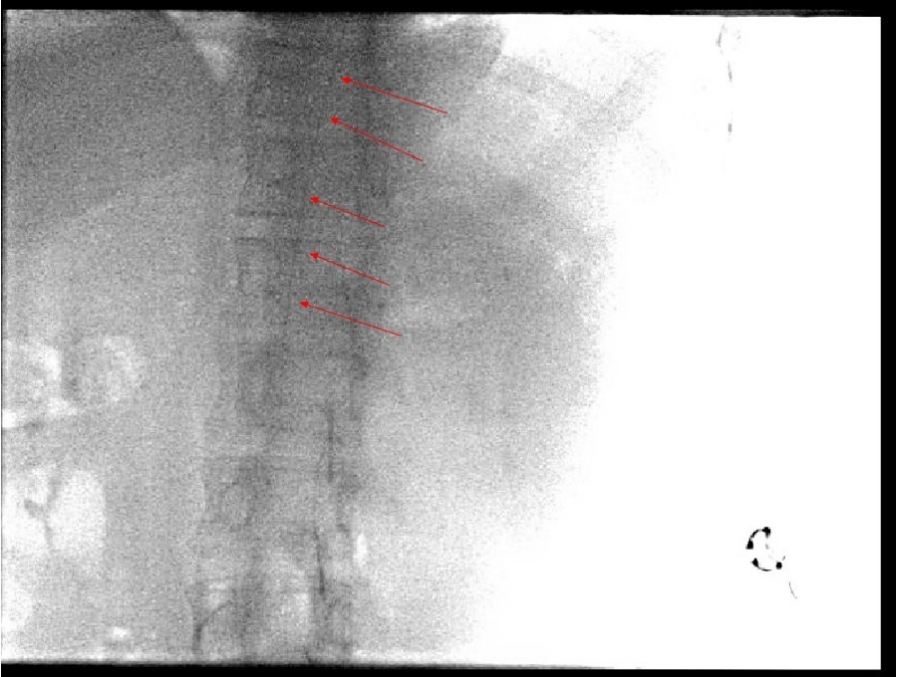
Posterior-Anterior Radiograph Showing Catheter in the Thoracic Aorta (Red Arrows) Coaxial 4.3 French Detachable Apollo Catheter and Marksman Microcatheter in the thoracic aorta.
